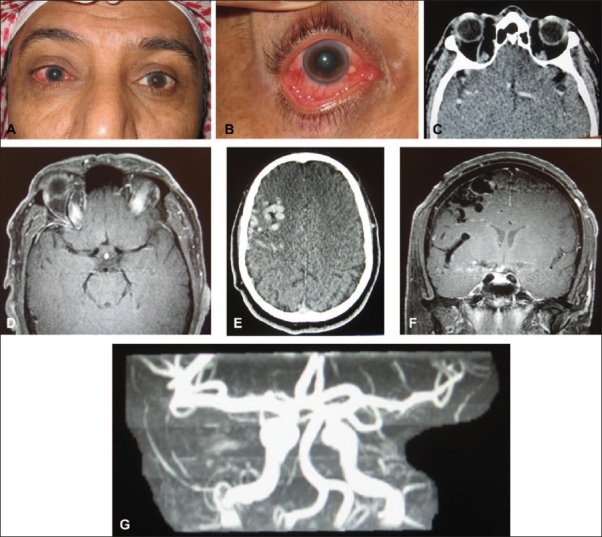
This 51-year-old man presented with right-sided proptosis, dilated pupil, elevated intraocular pressure and dilated episcleral vessels (A, B). CT scan and MRI revealed enlarged right-sided SOV (C, D) associated with cerebral signal void (E, F), suggestive of CCF. MRA confirmed presence of CCF (G)
